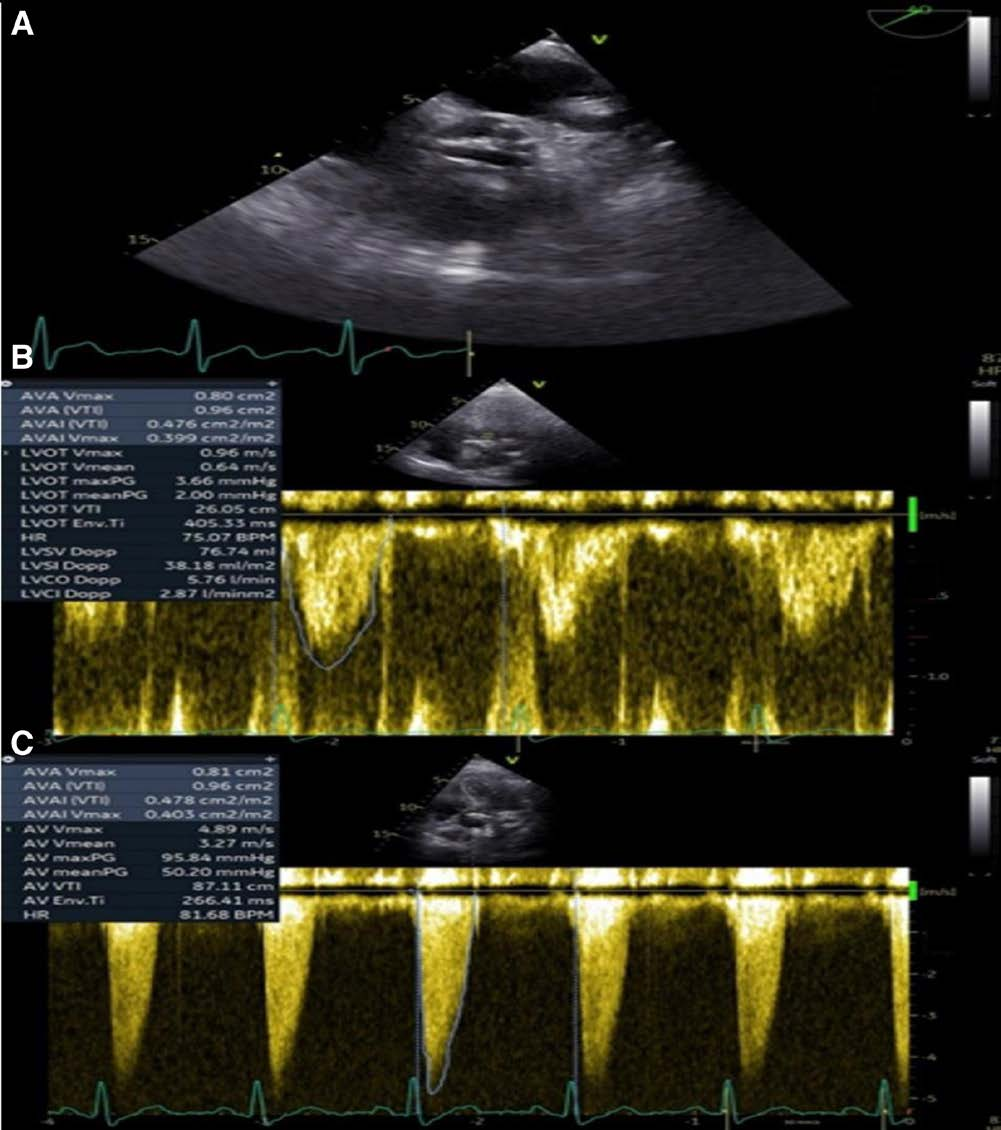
Severe aortic stenosis on transesophageal echocardiography (A), pulsed Doppler echocardiography during dobutamine loading focusing on blood flow in the left ventricular outflow tract (B), continuous Doppler echocardiography during dobutamine loading focused on the aortic valve (C).
